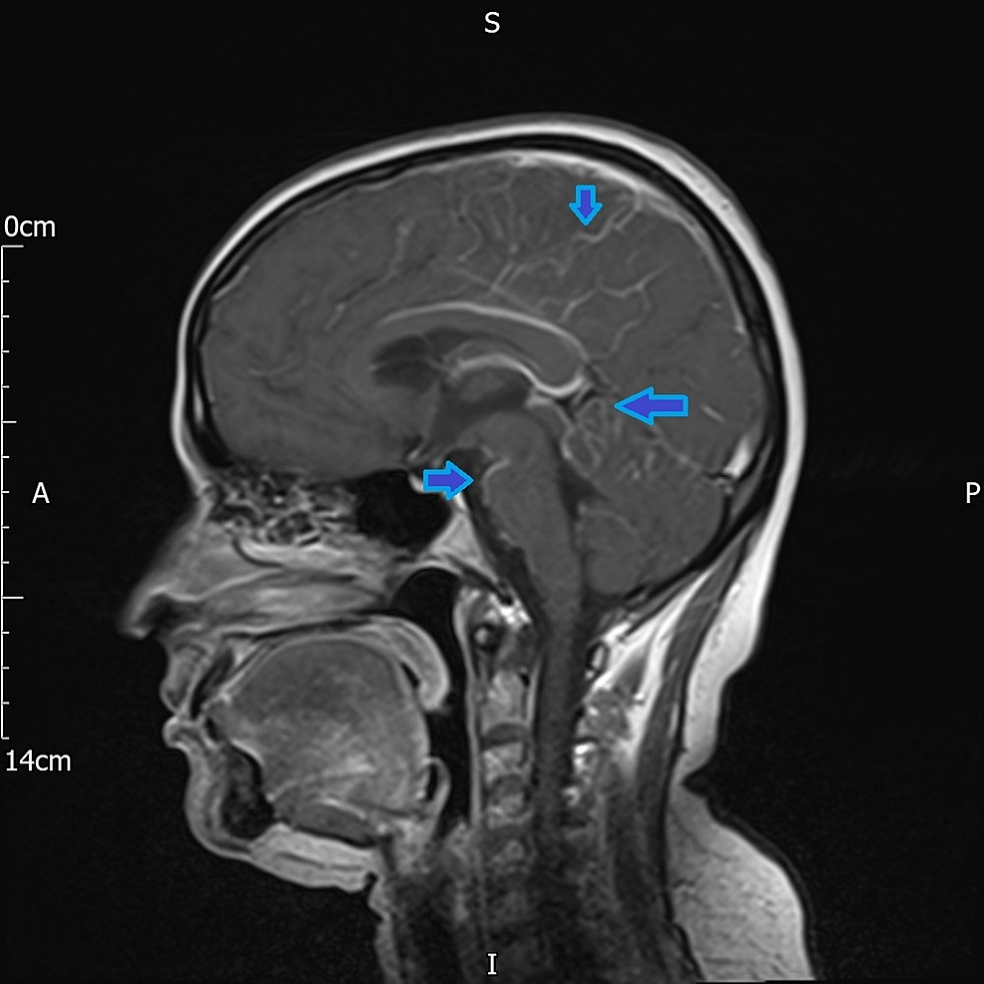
T1W Sagittal post-contrast MRI head showing leptomeningeal enhancement in the superior cerebellum.
